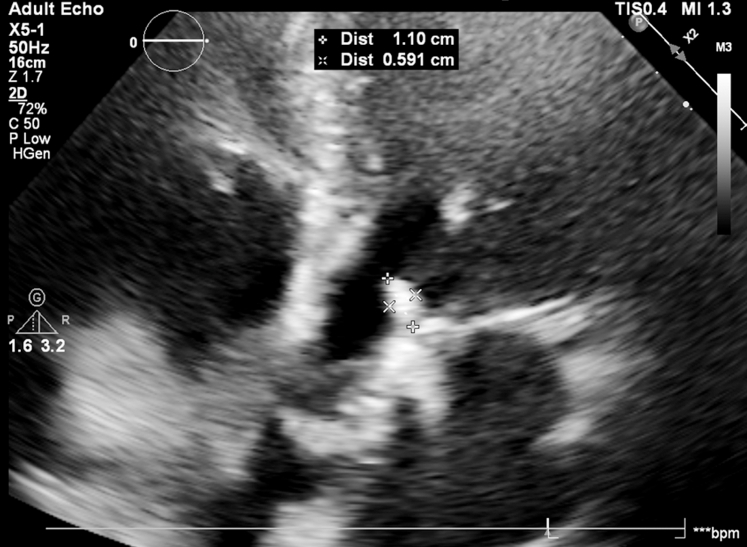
Frozen Image From the TTE of Patient 1 This image demonstrates a new echodensity that was not seen or appreciated on the patient's prior TTE. The echodensity was measured at 1.1 × 0.591 cm. TTE = transthoracic echocardiogram.
